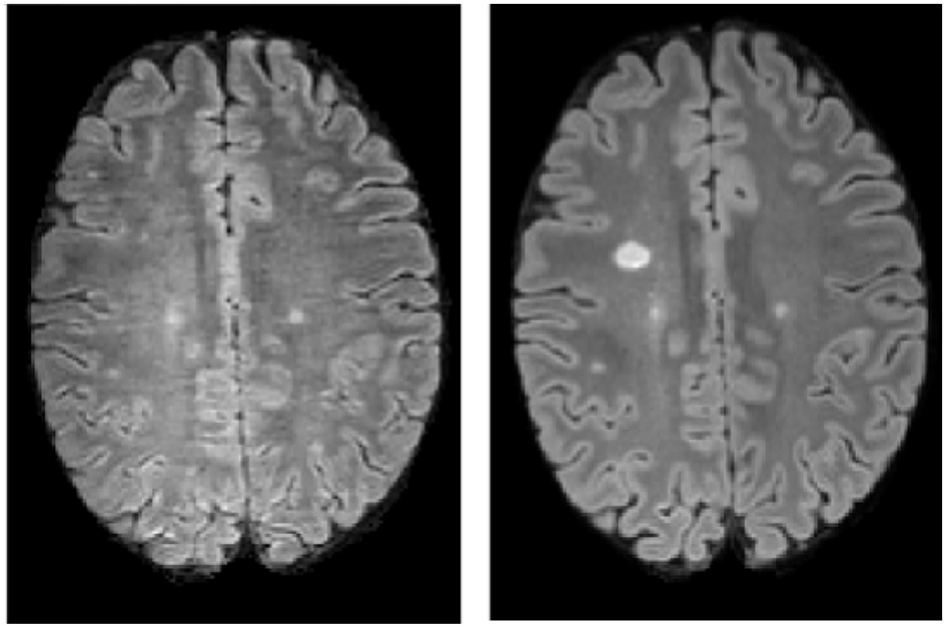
A pair of FLAIR acquisitions from a patient of the evaluation dataset experiencing a low quality baseline scan. (Left) Baseline FLAIR axial slice. (Right) Follow-up FLAIR axial slice.
